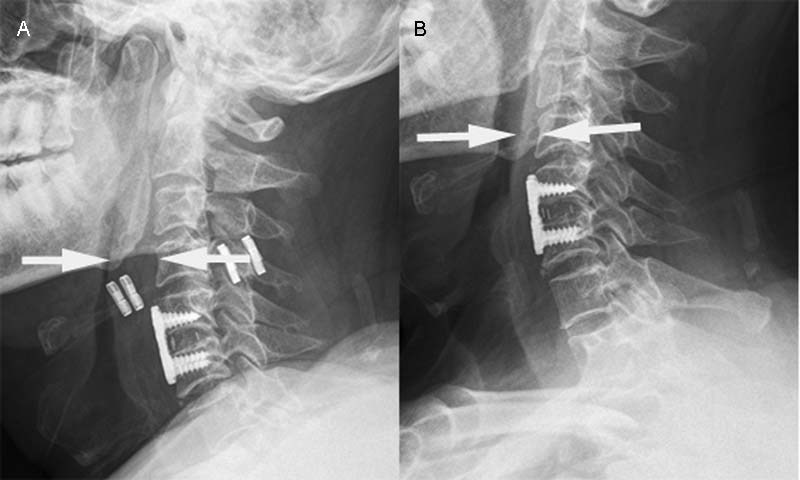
Postoperative X-rays of the cervical spine: (
